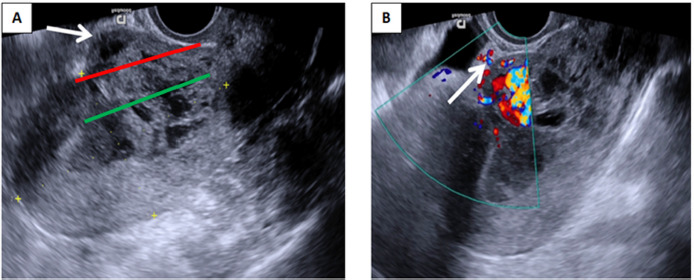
(A) Transvaginal grayscale ultrasound demonstrates heterogeneous mass inside the uterine cavity protruding anteriorly through the scar tissue (arrow). Both the serosal line (red line) and uterine cavity line (green line) are crossed. (B) Colour Doppler imaging reveals intense vascularity in the vesicouterine space (arrow), involving posterior wall of urinary bladder
